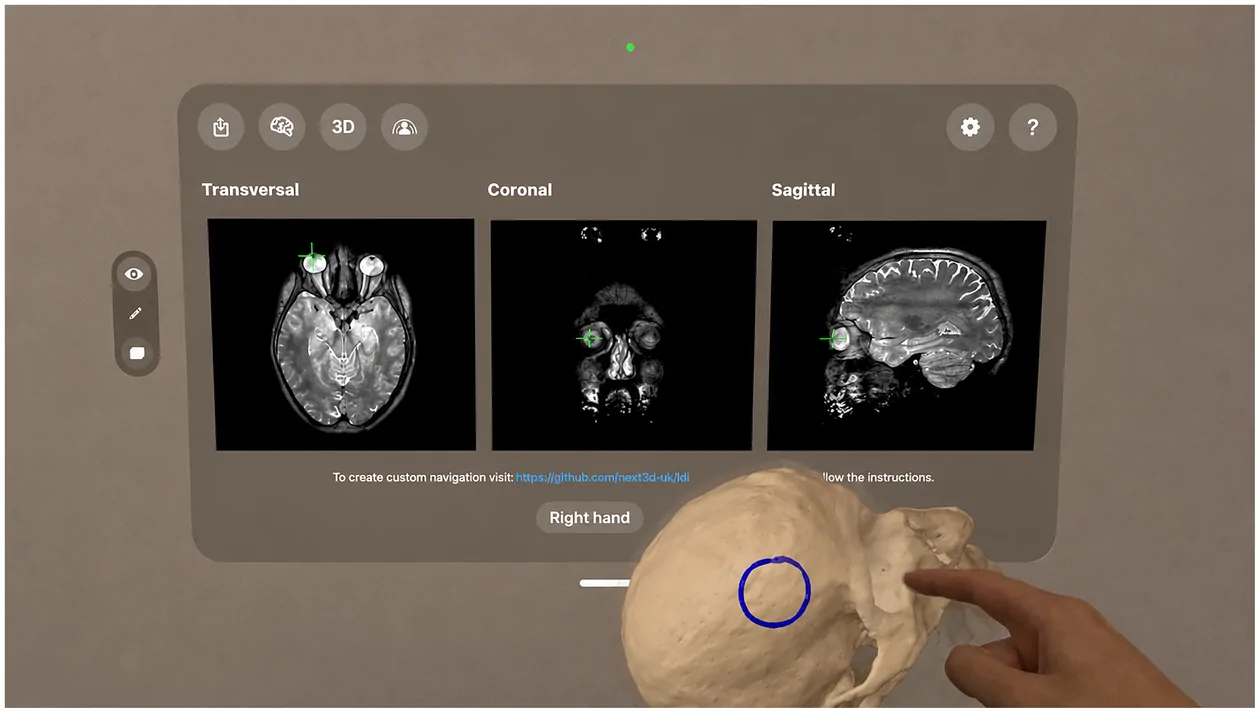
With the help of the navigation tool the surgeons can see inside the corresponding CT/MRI images the current position of the fingertip in the anatomical 3D model.
